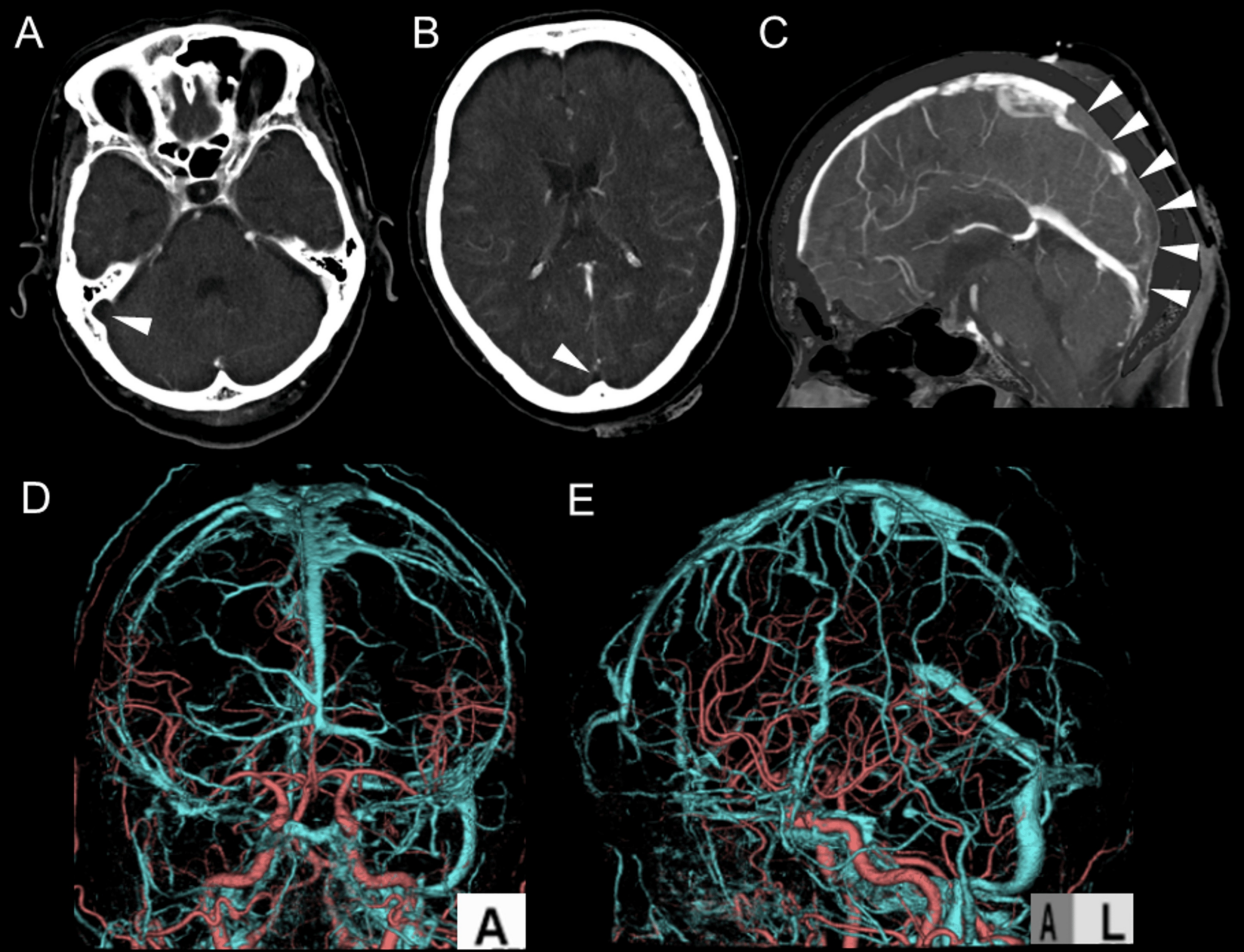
CTV (A, B, C), 3D CTA, and 3D CTV Fusion Images (D, E) Suspicion of thrombosis causing occlusion from the superior sagittal sinus to the right sigmoid sinus (arrow heads). CTV, computed tomography venography; CTA, computed tomography angiography
